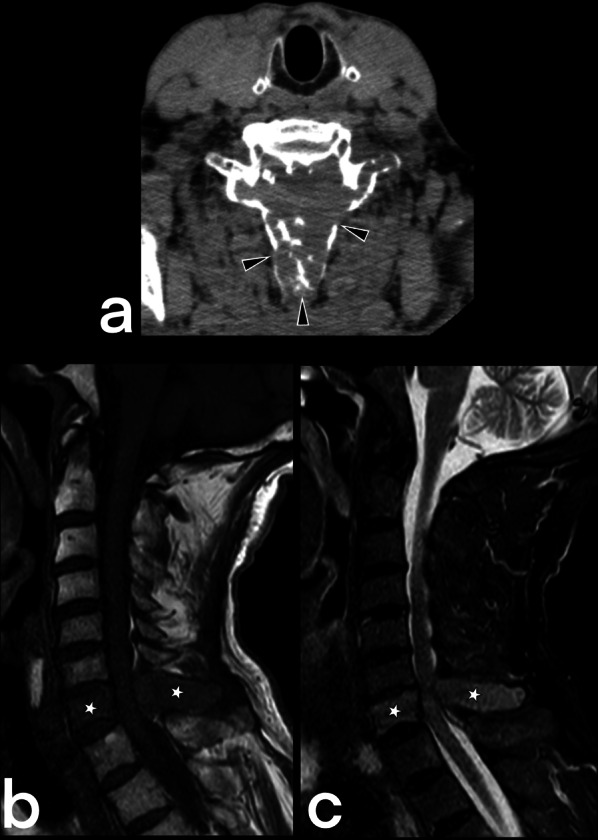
A 41-year-old male with pain in the posterior neck region. Non-contrast axial CT image (a) demonstrates destruction and bone erosion of the posterior spinous process of the C7 vertebra (arrowheads). Pre-contrast sagittal T1 weighted image (b) reveals diffuse decreased signal intensity within the c7 vertebral body and posterior spinous process. The lesion shows prominent diffuse contrast enhancement on sagittal (c) T1-weighted images (stars)
